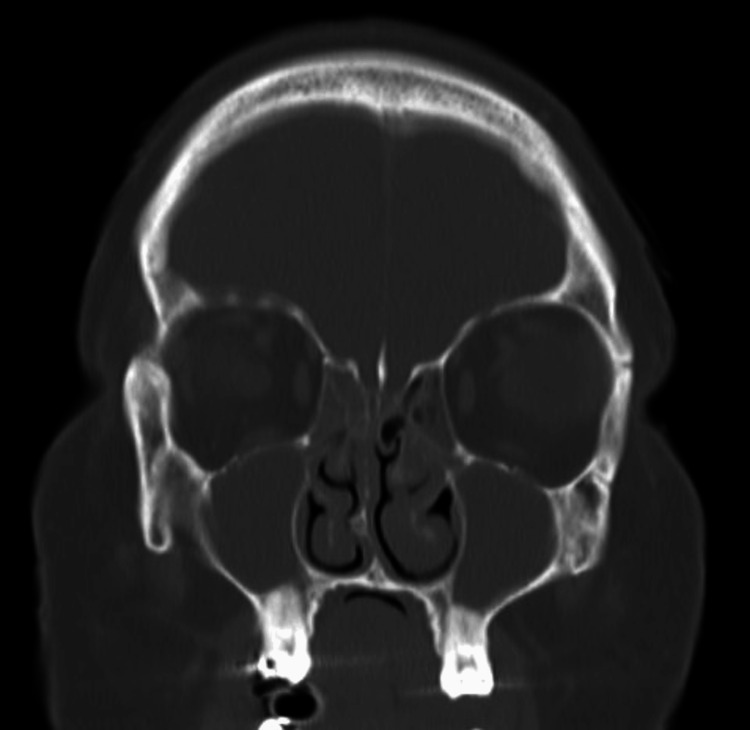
Contrast-enhanced CT Head with bone windows (coronal) demonstrates near-complete opacification of the bilateral maxillary sinuses and anterior ethmoid air cells.
